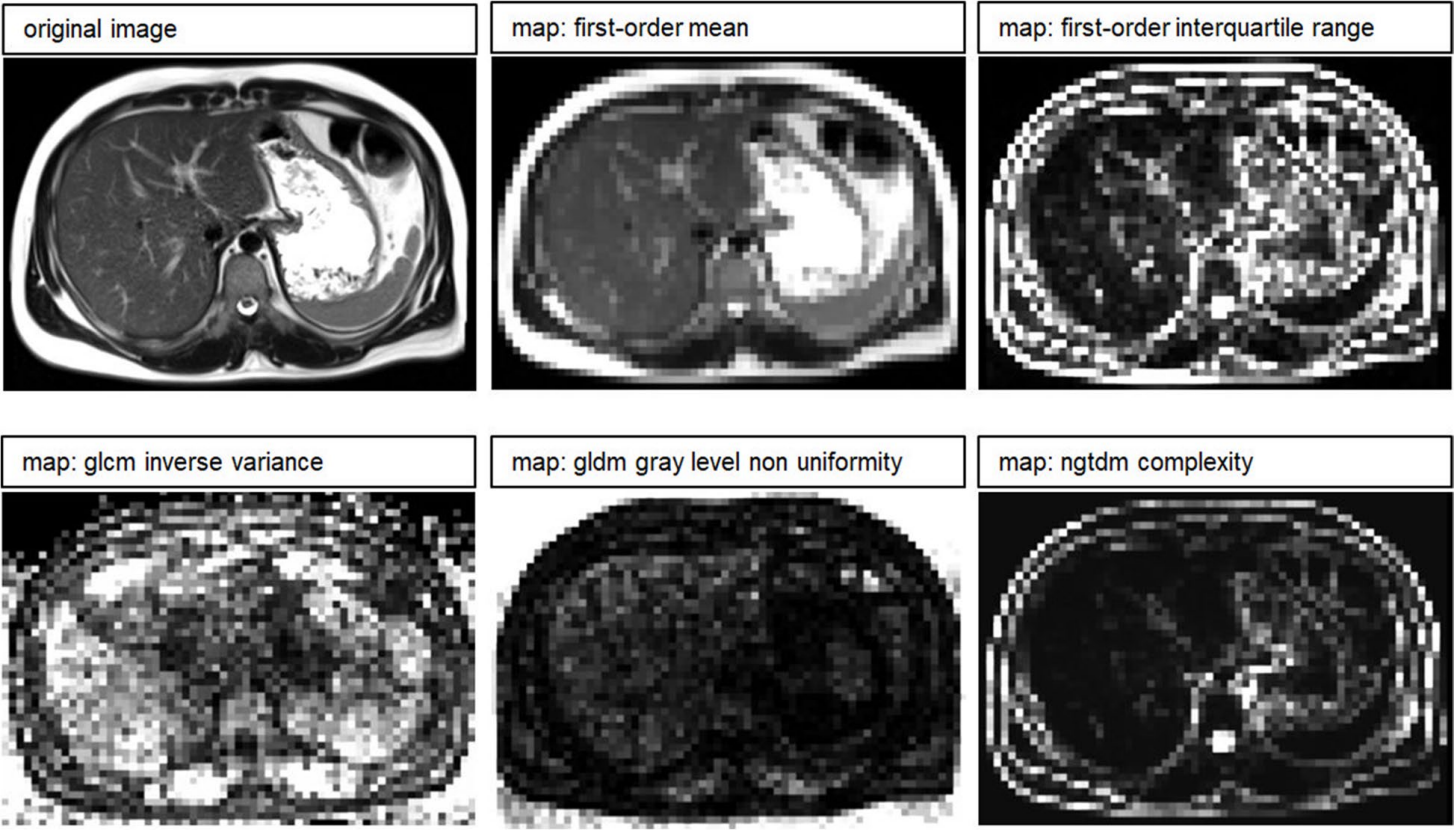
Exemplary feature maps. The original images were acquired on the 1.5-T scanner (scanner #3). The examples show the T2-weighted image and slices of the corresponding feature maps for first-order mean, first-order interquartile range, GLCM inverse variance, GLDM gray level non uniformity, and NGTDM complexity. Ninety-three features were included in our analysis, resulting in 93 parametric feature maps per patient for the T1-weighted and for the T2-weighted images
